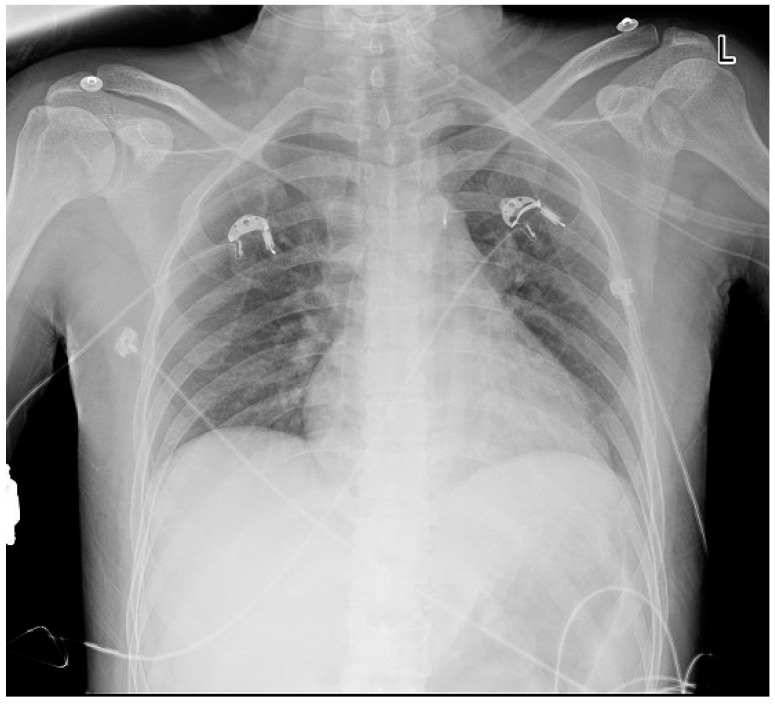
Chest X-ray shows significant cardiomegaly upon admission. The heart-to-chest ratio is 0.6.
